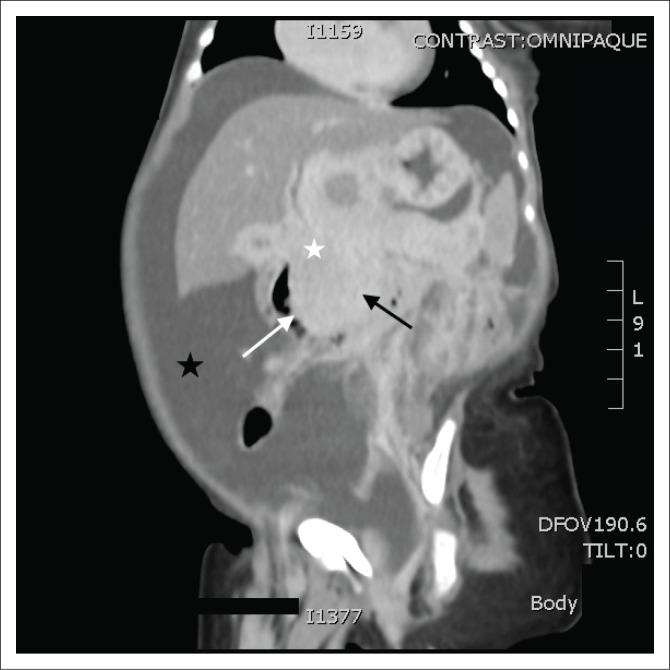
Coronal post-contrast computed tomography image demonstrating an ill-defined, enhancing mass in the head of the pancreas (black arrow), with a poor plane of separation from the D2 segment of the duodenum (white arrow) and peripancreatic extension to the porta hepatis (white star). Secondary findings of ascites (black star).
